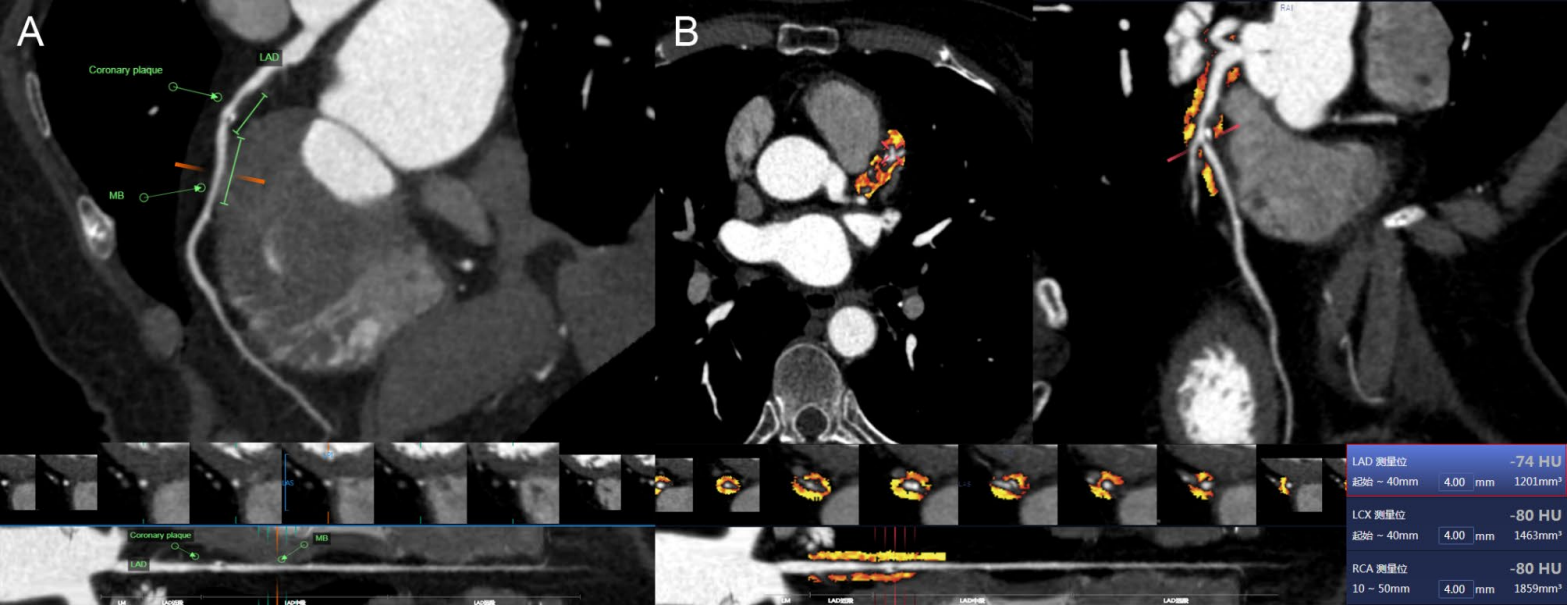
A 65-year-old male underwent CCTA. (a) The patient had an MB on the left anterior descending artery, and coronary artery stenosis covered by the MB was not obvious. The patient had high-risk plaque in the segment proximal to the MB. (b) The FAI_lesion_ value for the 40-mm segment around the coronary plaque proximal to the MB was − 74 HU (the red frame). *CCTA*: coronary computed tomography angiography, *MB*: myocardial bridge, *FAI*: fat attenuation index
